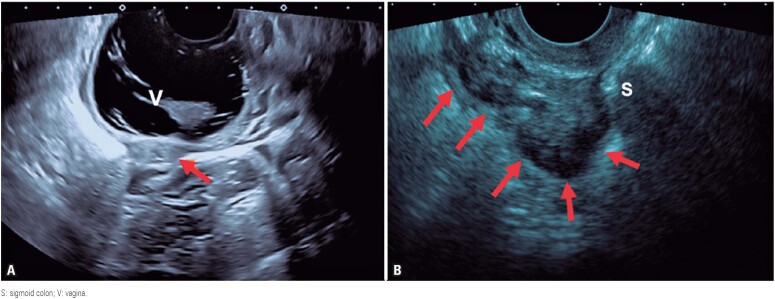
(A) A regular hypoechoic nodule (arrow) infiltrating the vaginal wall. The vagina was distended with sonographic gel for better delimitation of the lesion; (B) A transvaginal ultrasound of the sigmoid shows hypoechoic thickening of the bowel wall (arrows) infiltrating the muscular layer
